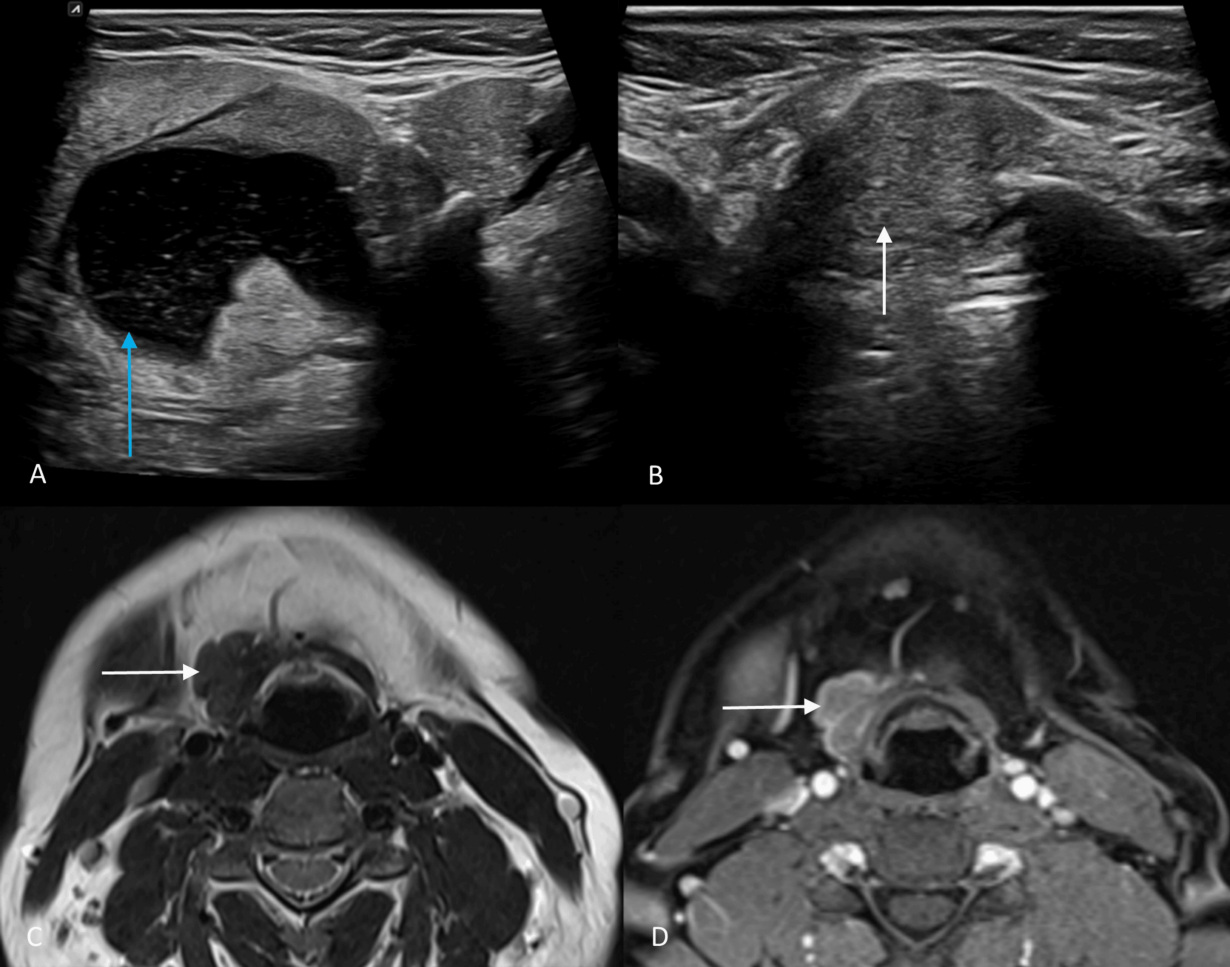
Ultrasound and MRI images of the suprahyoid and infrahyoid regions. (A and B) Gray-scale ultrasound images of the right suprahyoid and infrahyoid regions show both right submandibular cystic lesion (blue arrow) and infrahyoid solid lesion (white arrow). (C and D) Axial T2-weighted and post-contrast T1-weighted MRI of the neck shows a well-defined irregular solid lesion in the right infrahyoid region, appearing isointense on T2-weighted imaging (white arrow). It shows heterogeneous enhancement on post-contrast T1-weighted images (white arrow). MRI, magnetic resonance imaging
